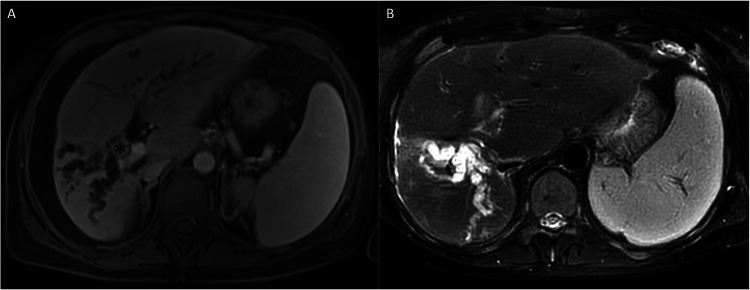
(A) Intraductal mass lesion (asterix) on contrast-enhanced T1 axial MRI series. (B) T2- weighted MRI image after PTBD to the left lobe and ascites resolution.
